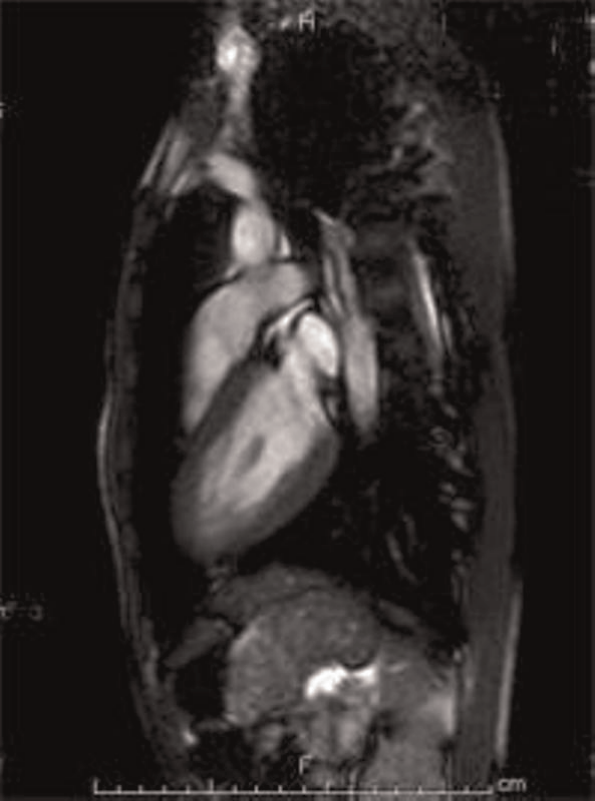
Gadlinium-enhanced cardiac MRI (TR: 3.57, TE:1.53) showed no enhancement on the myocardium.
